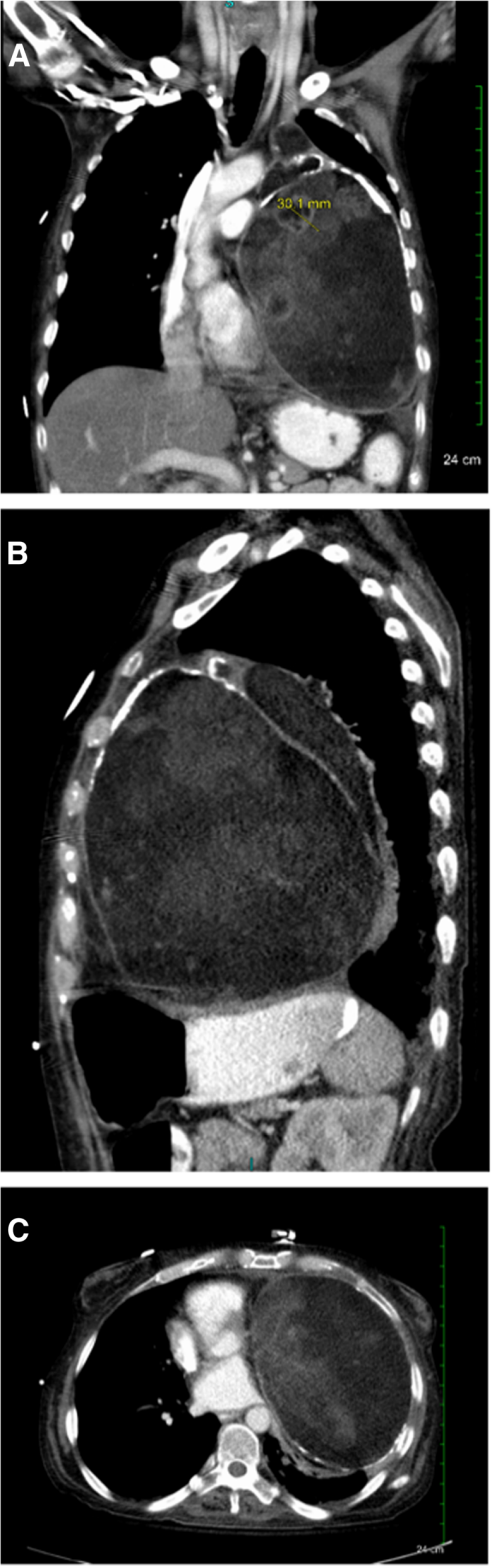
Contrast-enhanced-CT-imaging revealed a 20 cm well-circumscribed dense mass with areas of internal-enhancing-nodularity, creating mass effect on the heart, compressing the left heart and lung hilum. a AP b Sagittal c Transverse
